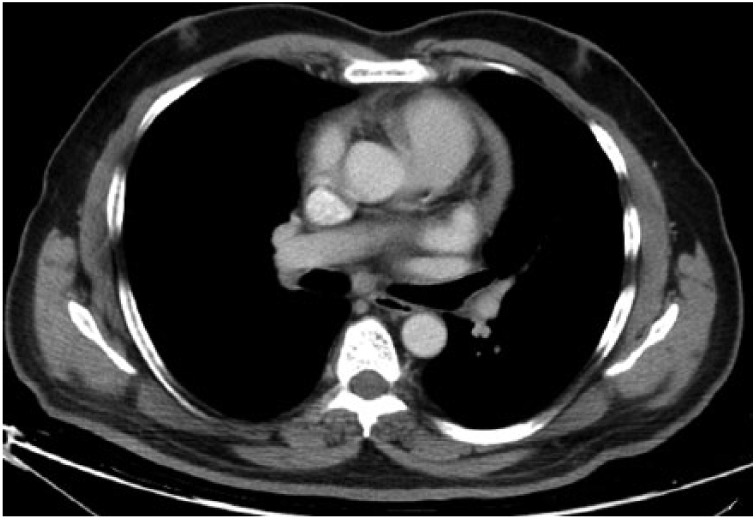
Control thorax HRCT with mediastinal window showing disappearance of hilar lymph nodes.
